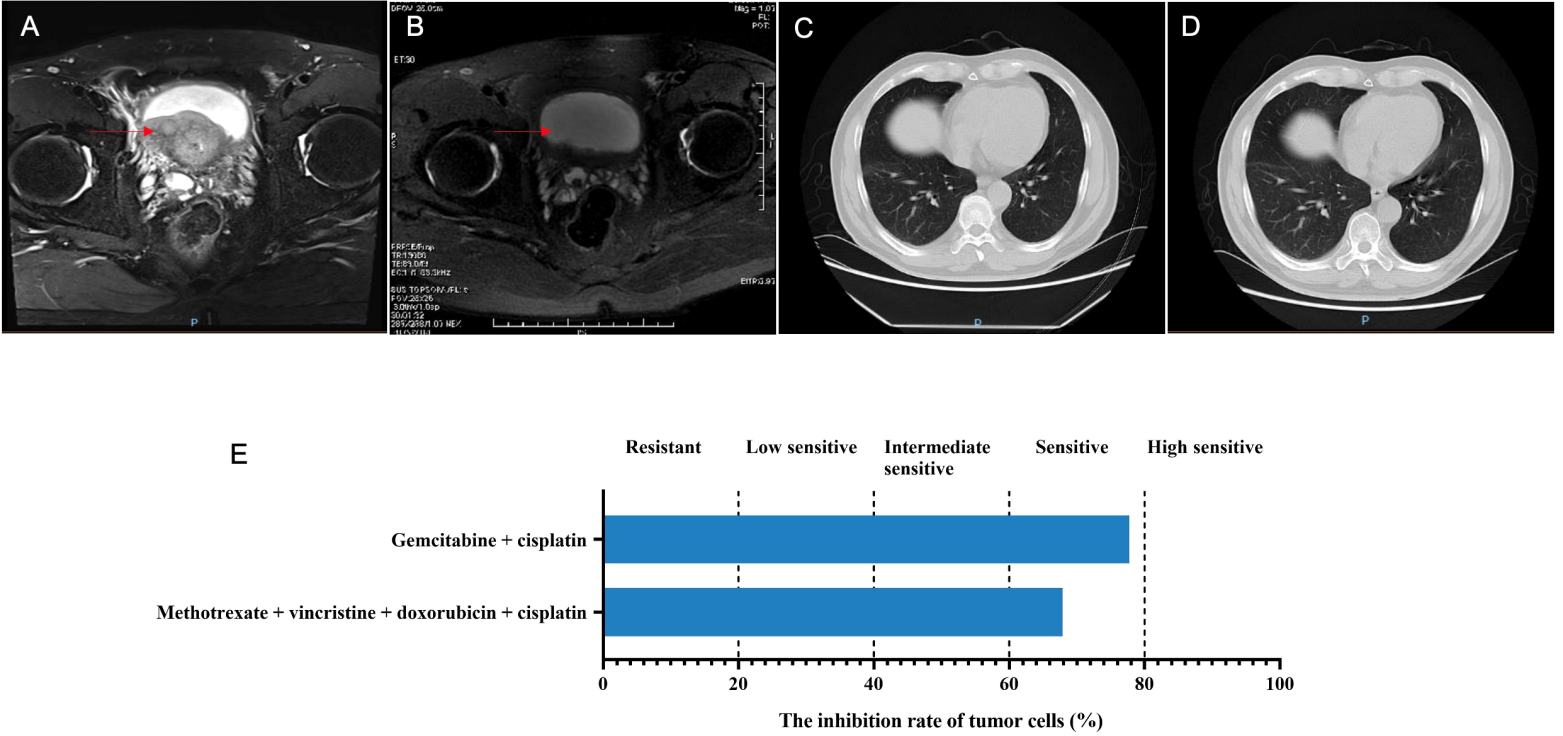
The disease changes of patient 1 based on MRI and CT scans, including initial diagnosis (A), post-neoadjuvant chemotherapy (B), 3 months after radical cystectomy (C), 9 months after radical cystectomy (D), as well as the PDO-based drug sensitivity testing results (E). The red arrows point to the tumor.
